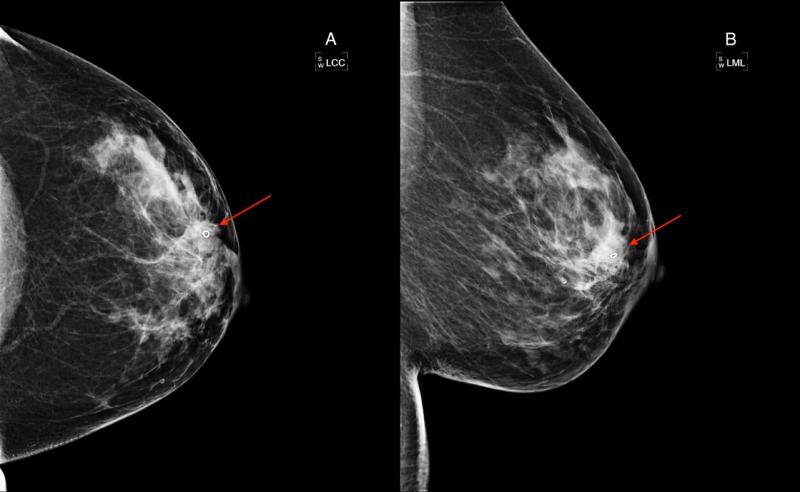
Diagnostic Mammogram following Ultrasound-guided Biopsy A diagnostic mammogram of the left breast after ultrasound guided biopsy is shown, and includes craniocaudal (A) and mediolateral (B) views. The marker indicates the biopsy site of a clinically palpable mass (red arrows). Note the unchanged fibroglandular component underlying the marker, effectively obscuring any defining features of malignancy.
